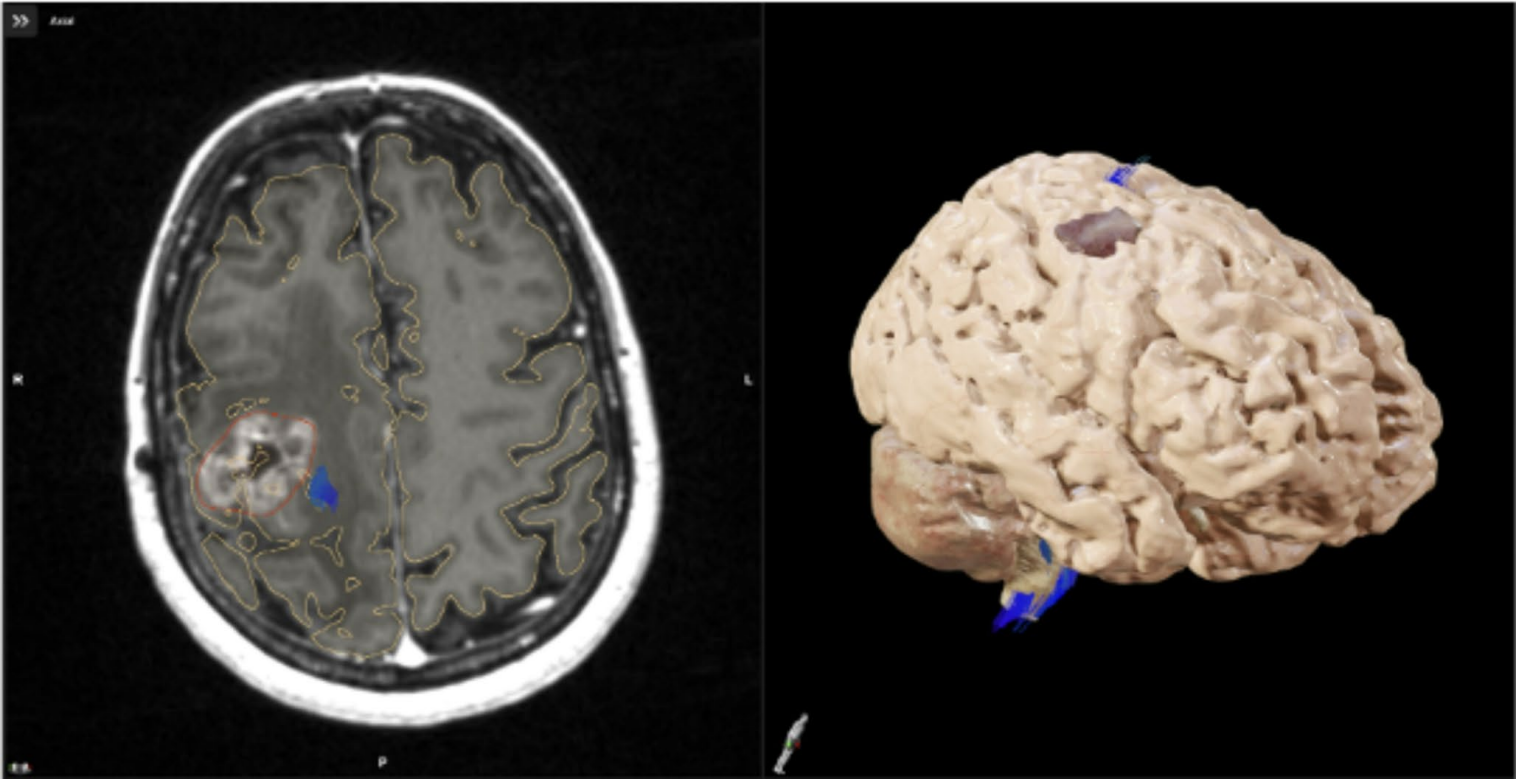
Screenshots demonstrating the planning sequences for the MagicLeap mixed reality visualisation using the BrainLab system. The screenshots (left: Magnetic Resonance Imaging (MRI) with auto-segmentation of cerebral volumes, tumour volume, and fibre tract bundles; right: 3D reconstruction resulting from A). Demonstration video available from these sequences (Supplementary materials)
